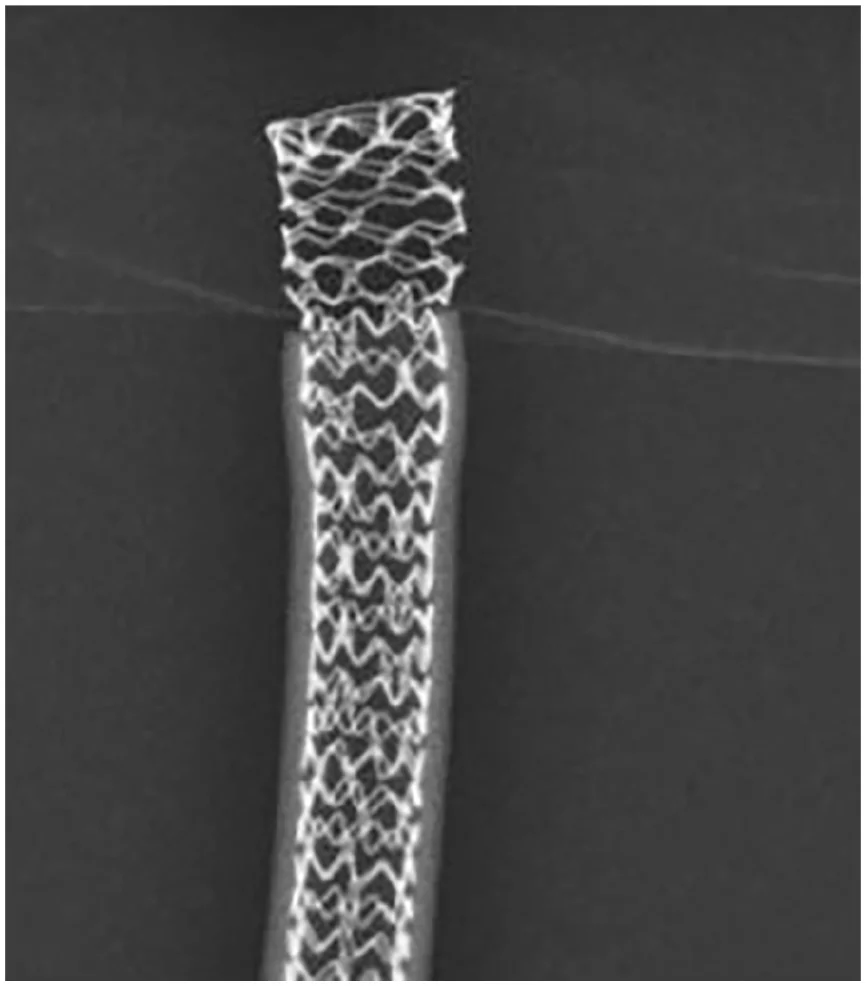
BeGraft stent explanted after in situ laser fenestration and fatigue up to 50 million cycles. The digital radiography shows the same structural changes of the endoskeleton observed after the flaring process without deterioration after 50 million cycles.
